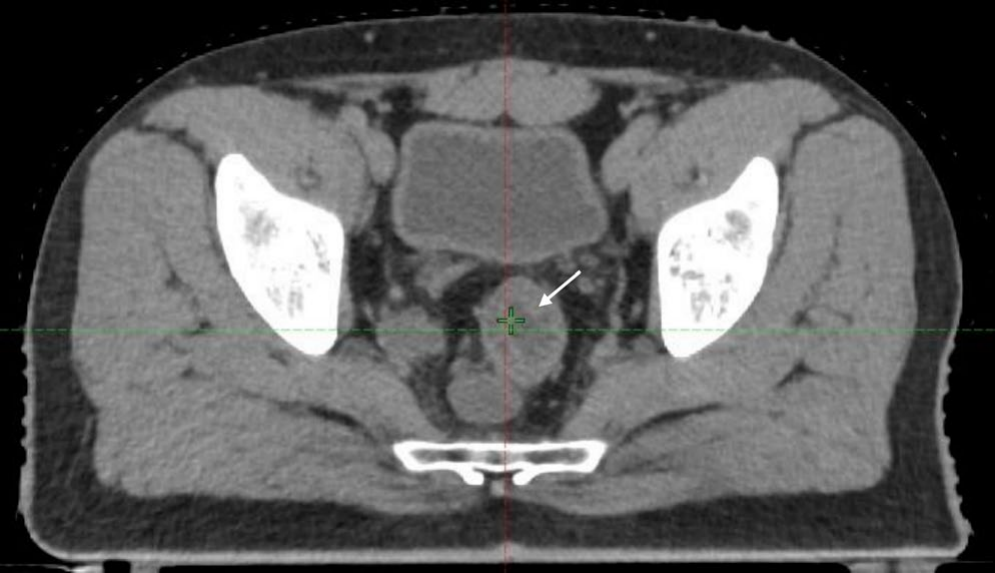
Contrast‐enhanced computed tomography of abdomen and pelvis showing heterogenously enhanced irregular circumferential thickening of anorectal wall with perirectal fat stranding (small white arrow) and multiple enlarged mesocolic and inguinal lymph nodes.
